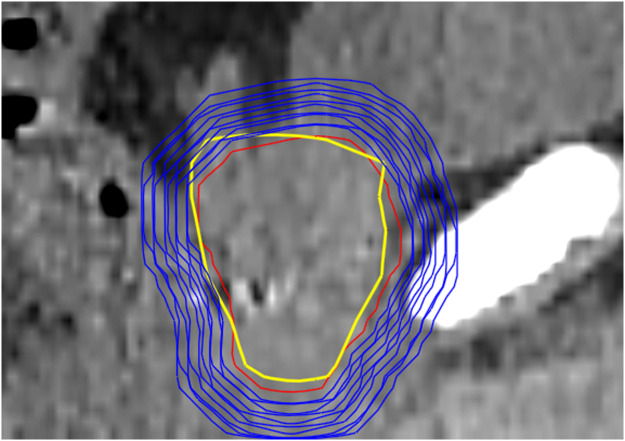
Sagittal visualization of initial cone beam computed tomography prostate clinical target volume (red), different planning target volume margins (blue), and verification cone beam computed tomography prostate clinical target volume (yellow) for an example cone beam computed tomography.
